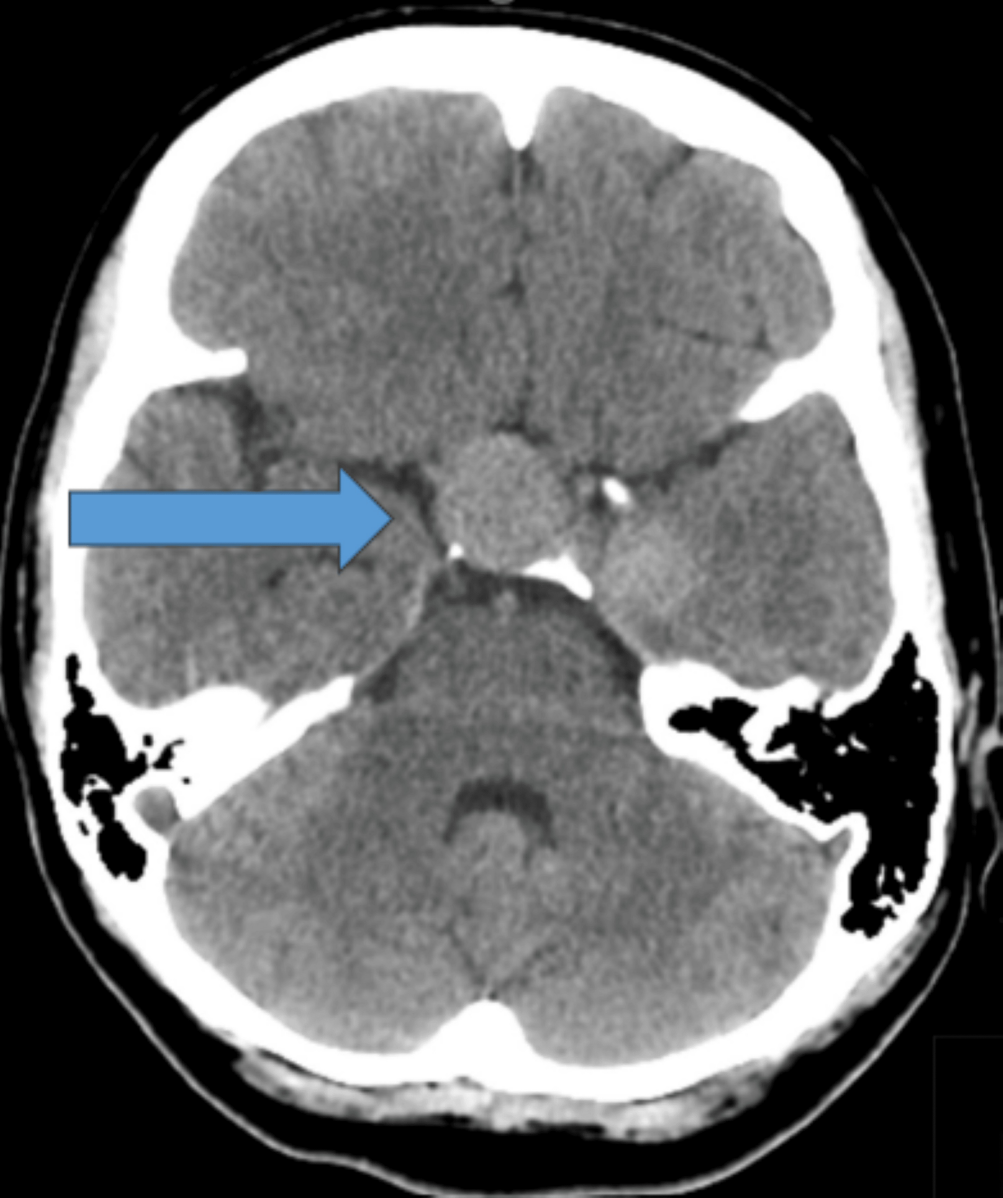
Axial Plane CT Scan Showing Hyperdense Pituitary Fossa (Blue Arrow). CT: computed tomography
